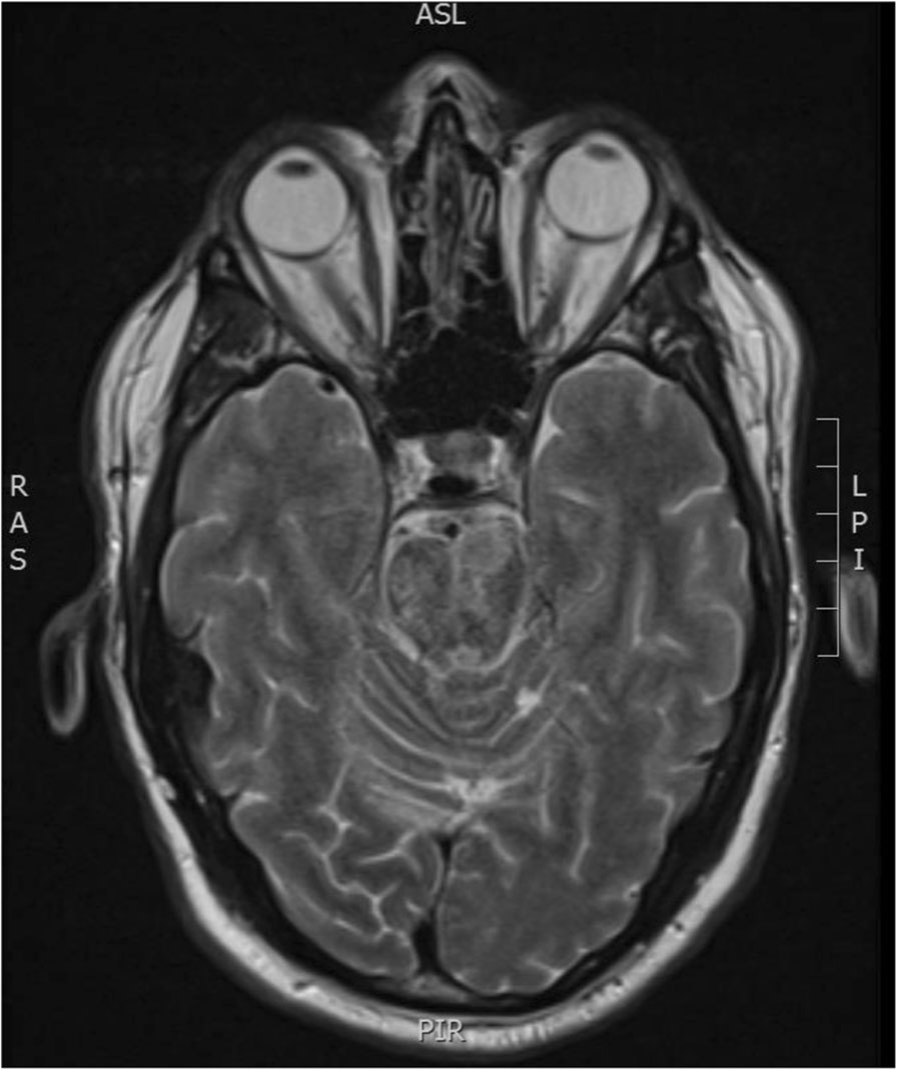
MRI brain showing bilateral midbrain infarcts
